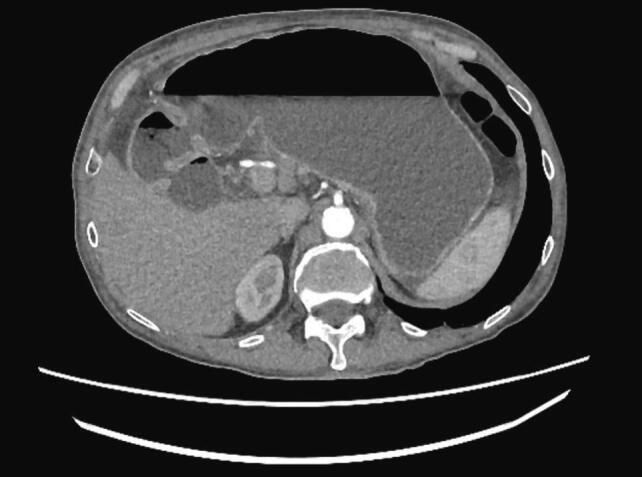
Dilated stomach with a large air-fluid level showed on abdominal computed tomography (CT).
